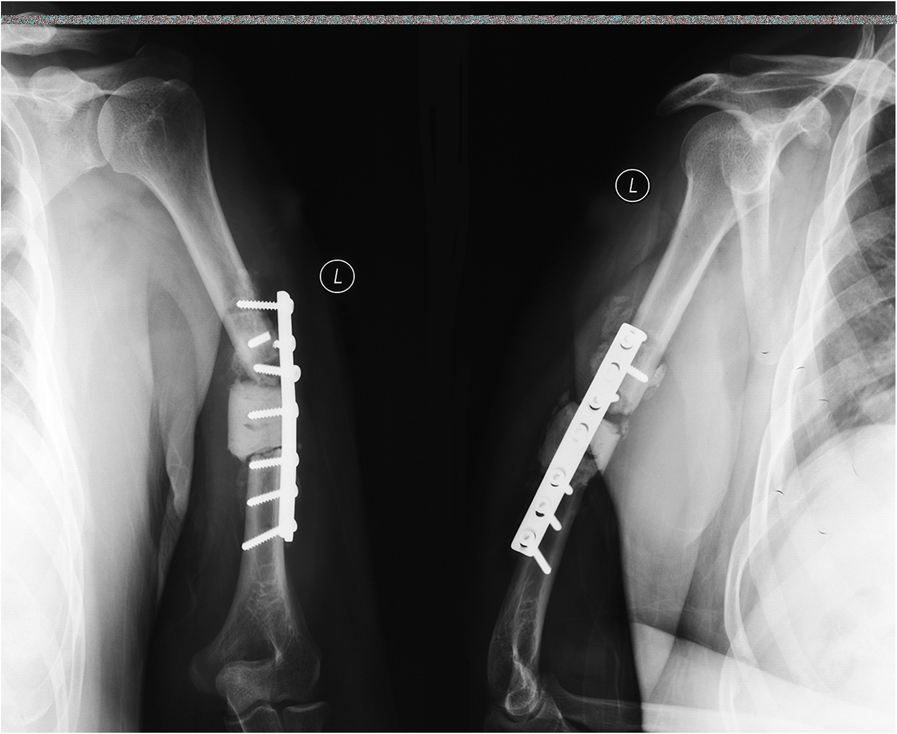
The X-ray film taken 5 months after the initial operation showed plate fixation failure, and revision surgery was performed
